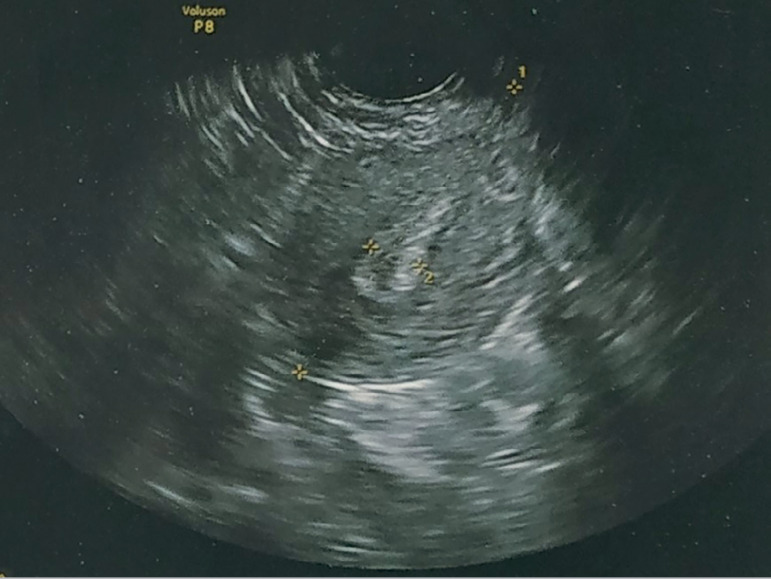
Baseline ultrasound before OS.
